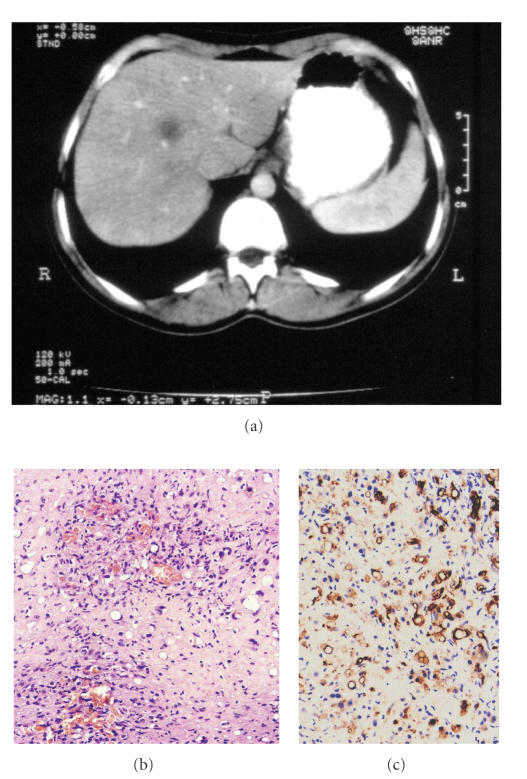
(a) Abdominal computed tomography showing an hypodense lesion in the liver. (b) Tumor composed of fibrous stroma with myxohyaline areas
